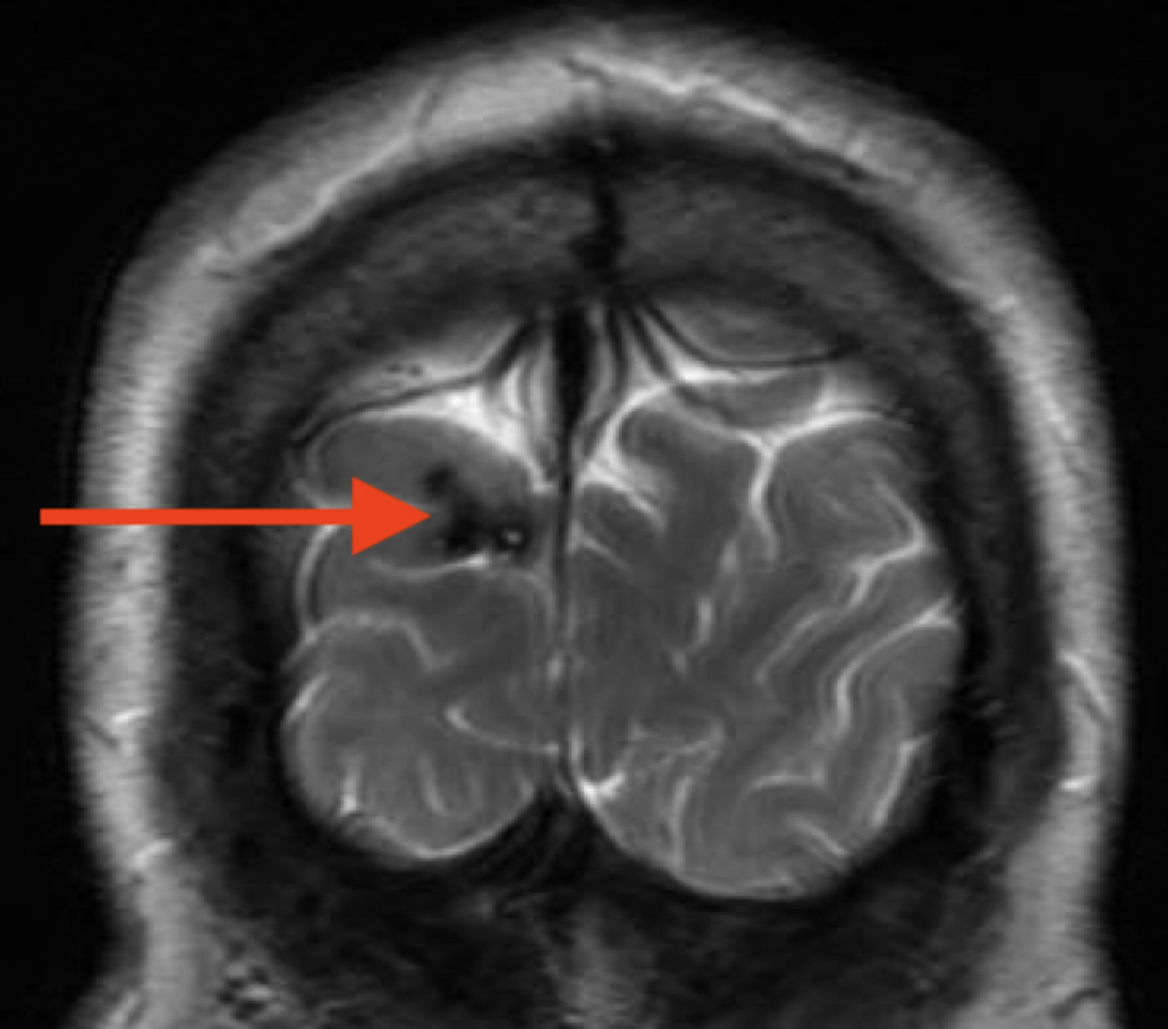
T2 MRI showing hemosiderin deposits in the right occipital lobe associated with cavernoma.
